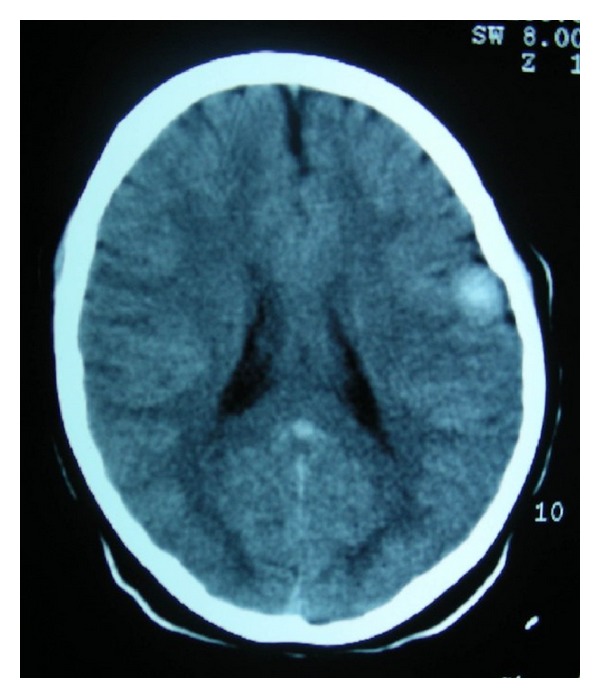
Preoperative axial CT scan showing a well-circumscribed superficial nodular hyperdense mass measuring 1.8 × 1.6 cm in the left frontoparietal lobe with remodeling of the inner table of the skull.
